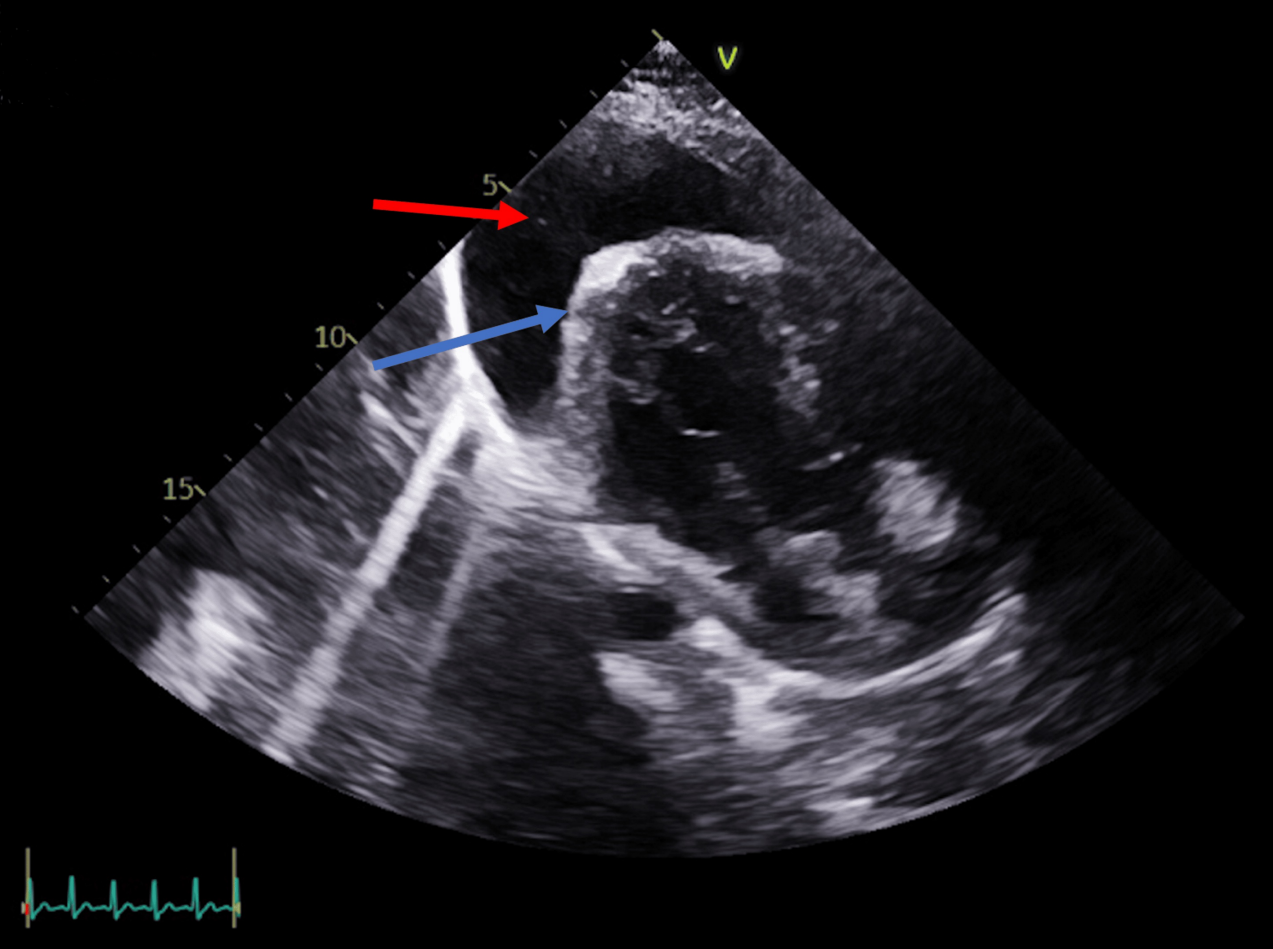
Echocardiogram showing large pericardial effusion. Pericardial effusion (red arrow). Pericardium (blue arrow). Large pericardial effusions, such as this one, can precipitate cardiac tamponade, which compresses the heart and results in decreased cardiac filling, thereby impairing systemic circulation.
